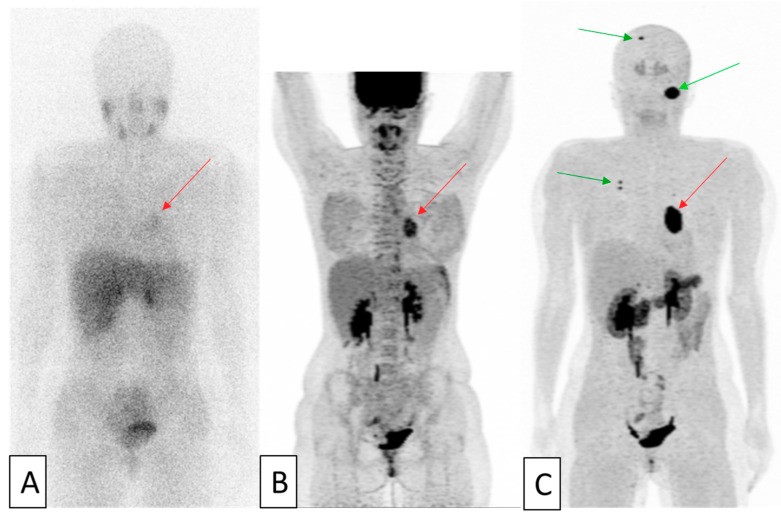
An example of 37-year-old patient with pluri-metastatic paraganglioma. MIP (maximum intensity projections) images of the realized 123MIBG-scintigraphy (A), FDG-PET (B) and FDOPA-PET (C) are shown. The subject has a mediastinal lesion, barely seen on 123MIBG-scintigraphy and clearly positive with the others tracers (red arrows). Pulmonary and skull lesions (green arrows) were only visible on FDOPA-PET. Images courtesy of Dr C. Ansquer © Catherine Ansquer
